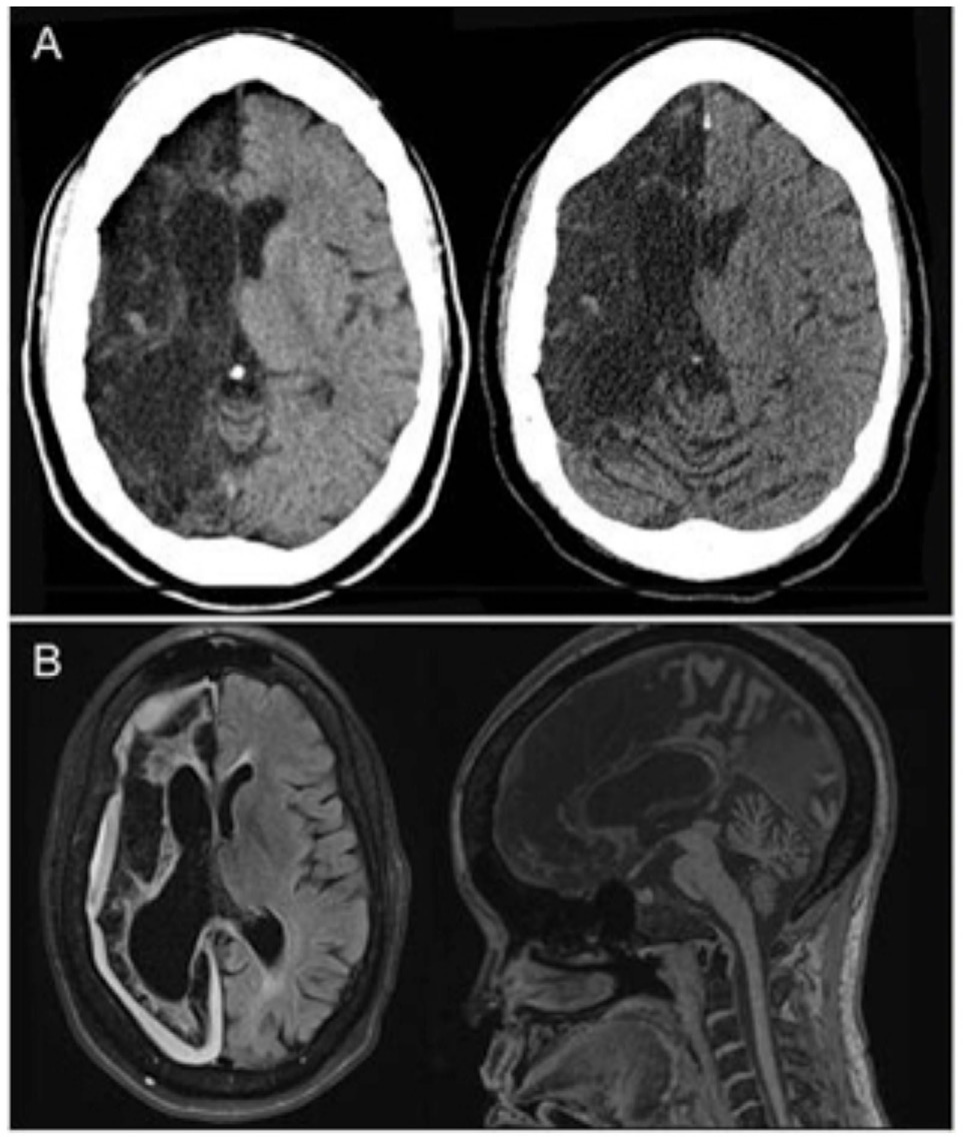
(A) Non-contrast head CT (*transverse views*) shows chronic right anterior cerebral artery (ACA) and middle cerebral artery (MCA) territory encephalomalacia with sparing of a small portion of the medial right frontal lobe, without evidence of bleed or new territory of acute infarction. (B) Both transverse and midsagittal MRI views obtained during an identical clinical episode from 3 years prior were likewise unremarkable for acute pathology.
